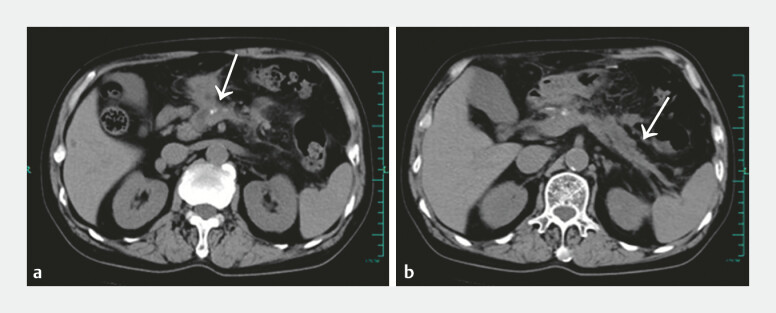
Abdominal enhanced CT images.
